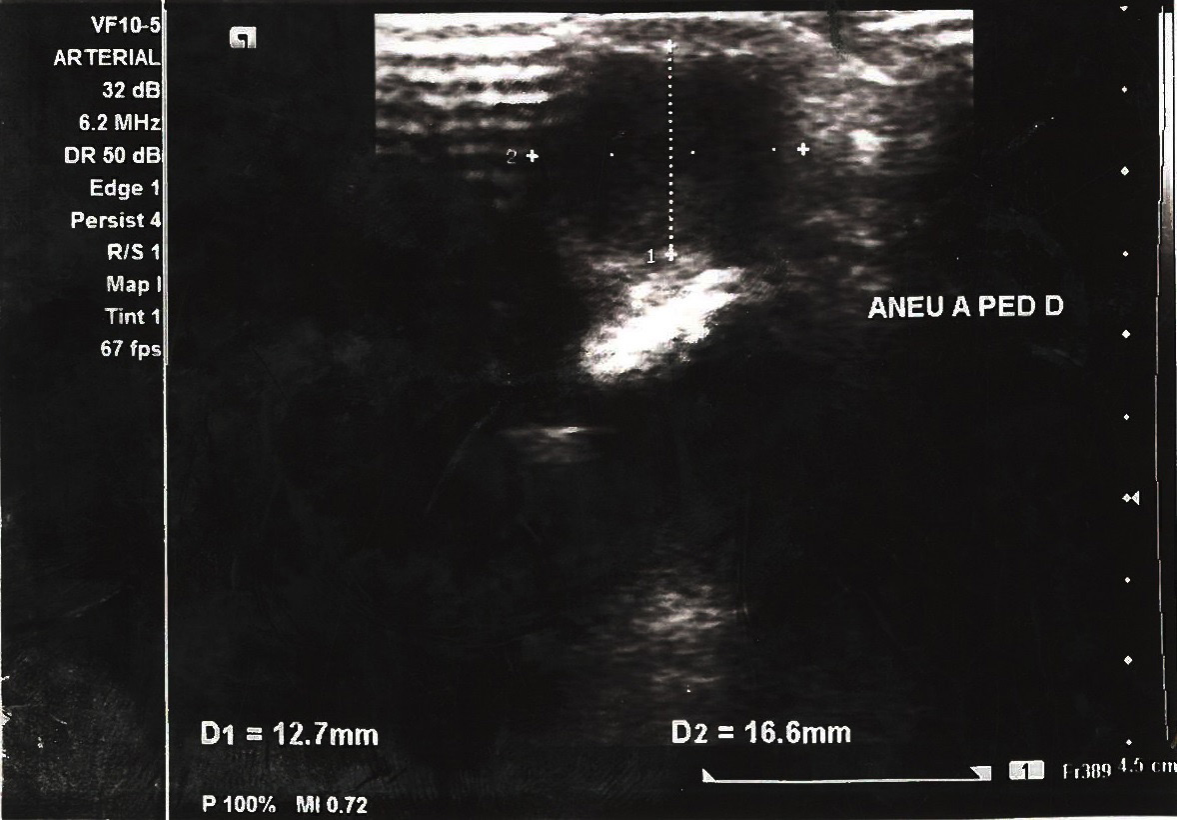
Aneurysmal dilatation of the dorsal artery of the right foot seen on ultrasound.
